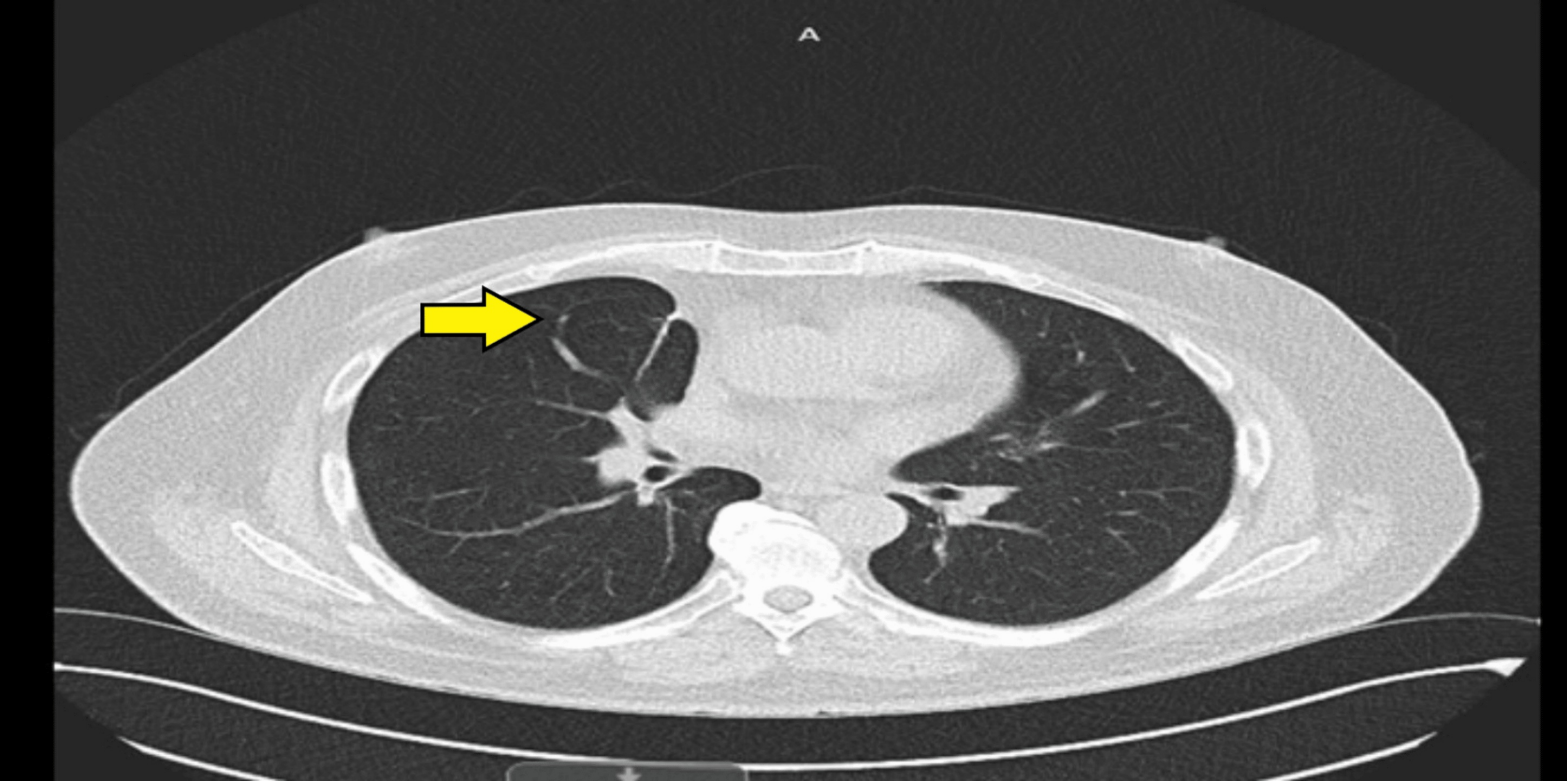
Axial CT scan of the chest demonstrating postsurgical changes in the medial segment of the right middle lobe following VATS with wedge resection (yellow arrow) CT: computed tomography; VATS: video-assisted thoracoscopic surgery
